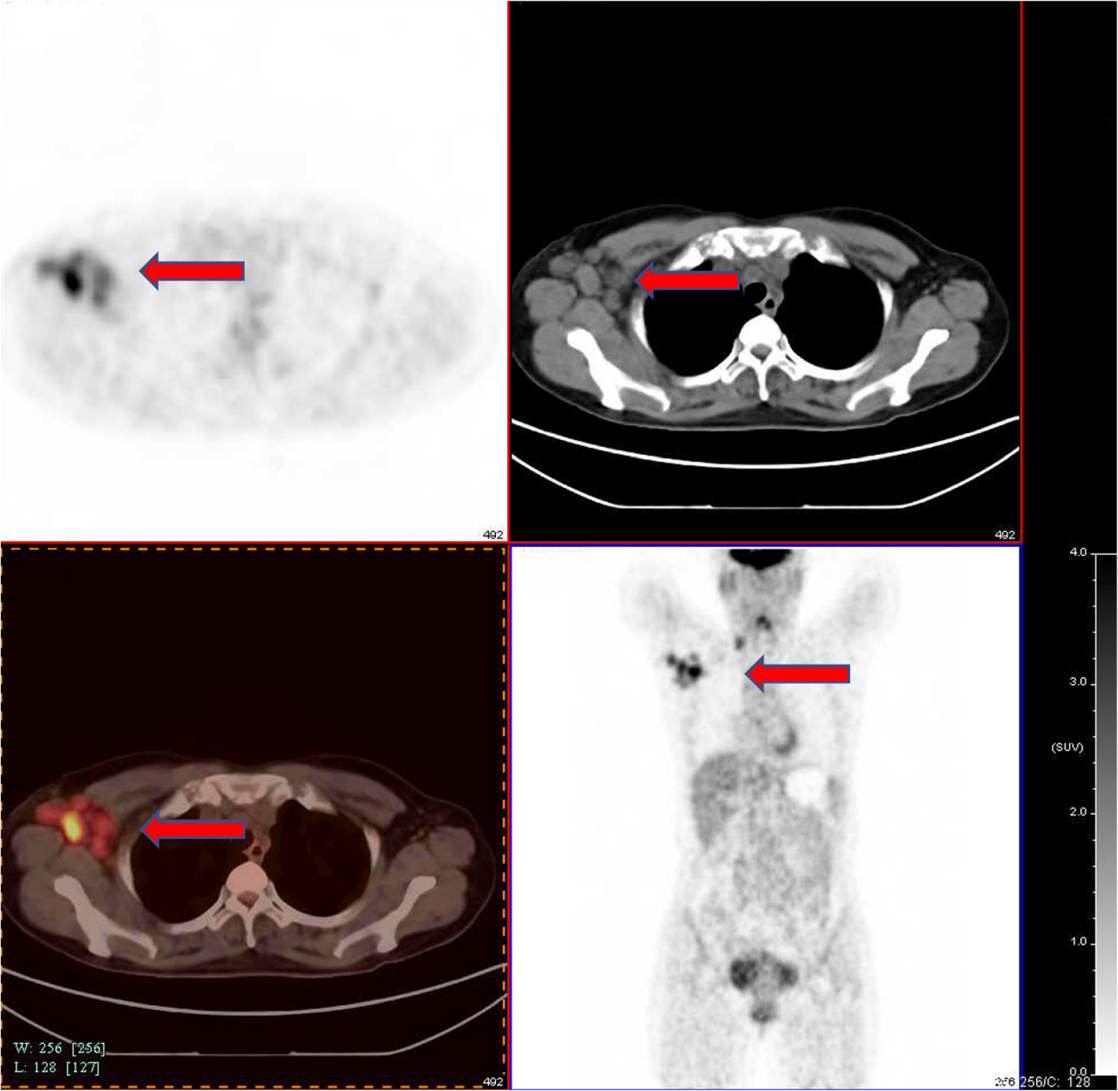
PET-CT before axillary surgery. The initial staging showed multiple lesions with significantly increased FDG intensity presenting in the right side axilla
